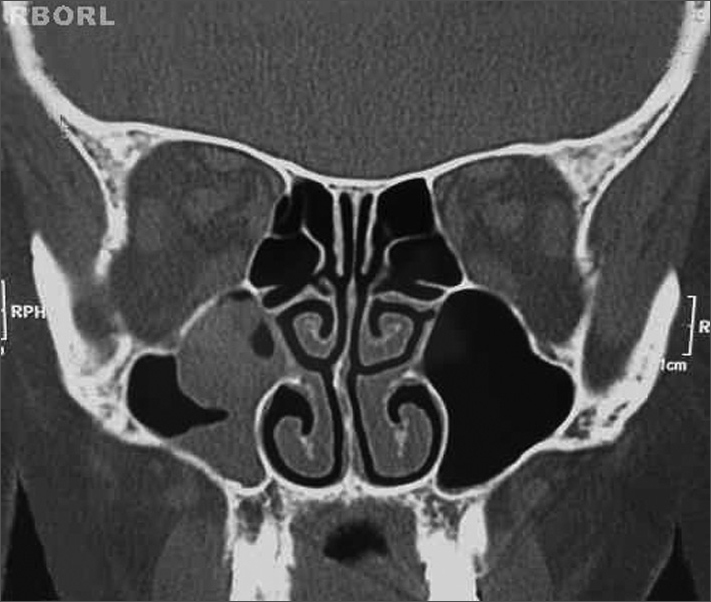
Oncocytic papilloma. Coronal CT showing an opacified right maxillary sinus.
